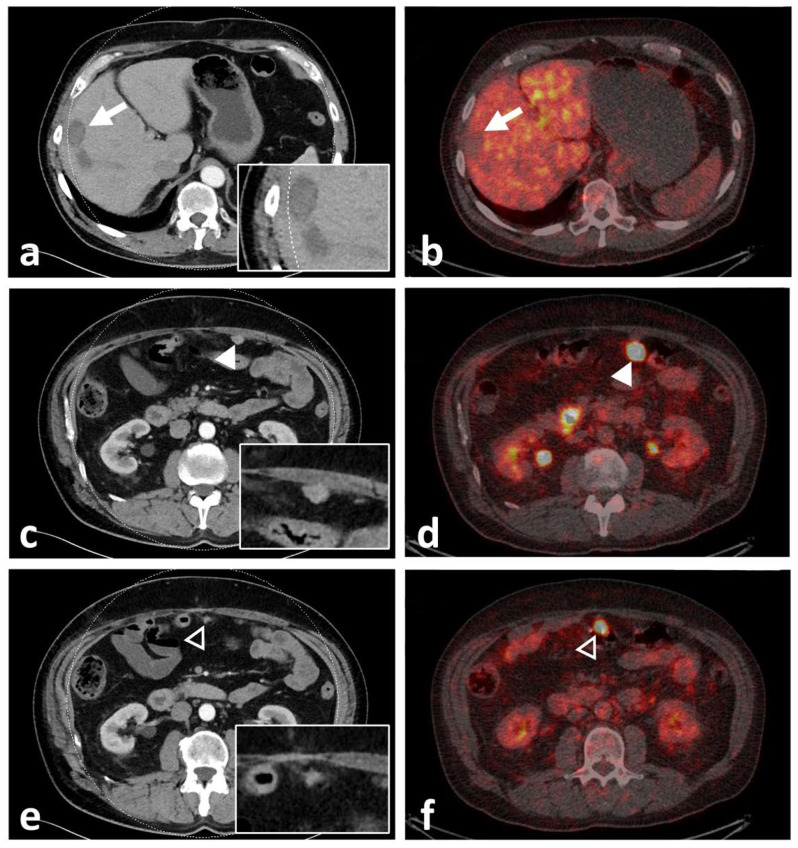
Computed tomography (CT) with inserted magnifications (left) and axial fused PET/CT images (right) of participant 9, demonstrating lack of uptake of ^68^Ga-NeoBOMB1 in a mildly enhancing liver metastasis (white arrow, (a,b)) and two omental metastases with significant tracer uptake (c,d) [white arrowhead] and (e,f) [black arrowhead]). The latter lesion was missed upon initial staging by CT.
